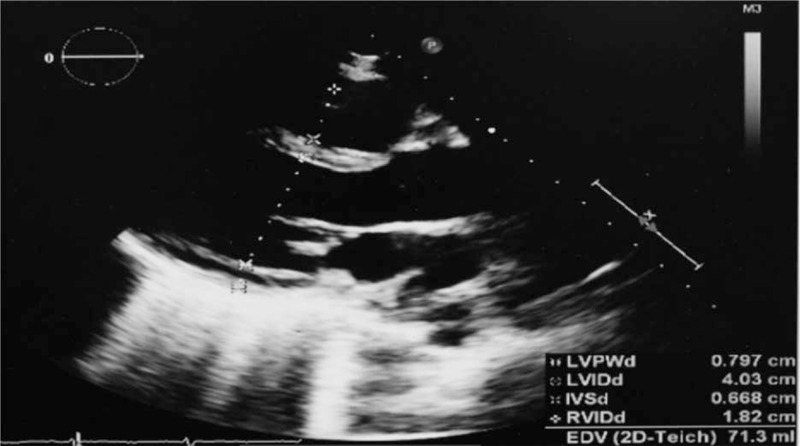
Follow-up echocardiography after the first cycle of IA induction chemotherapy.
